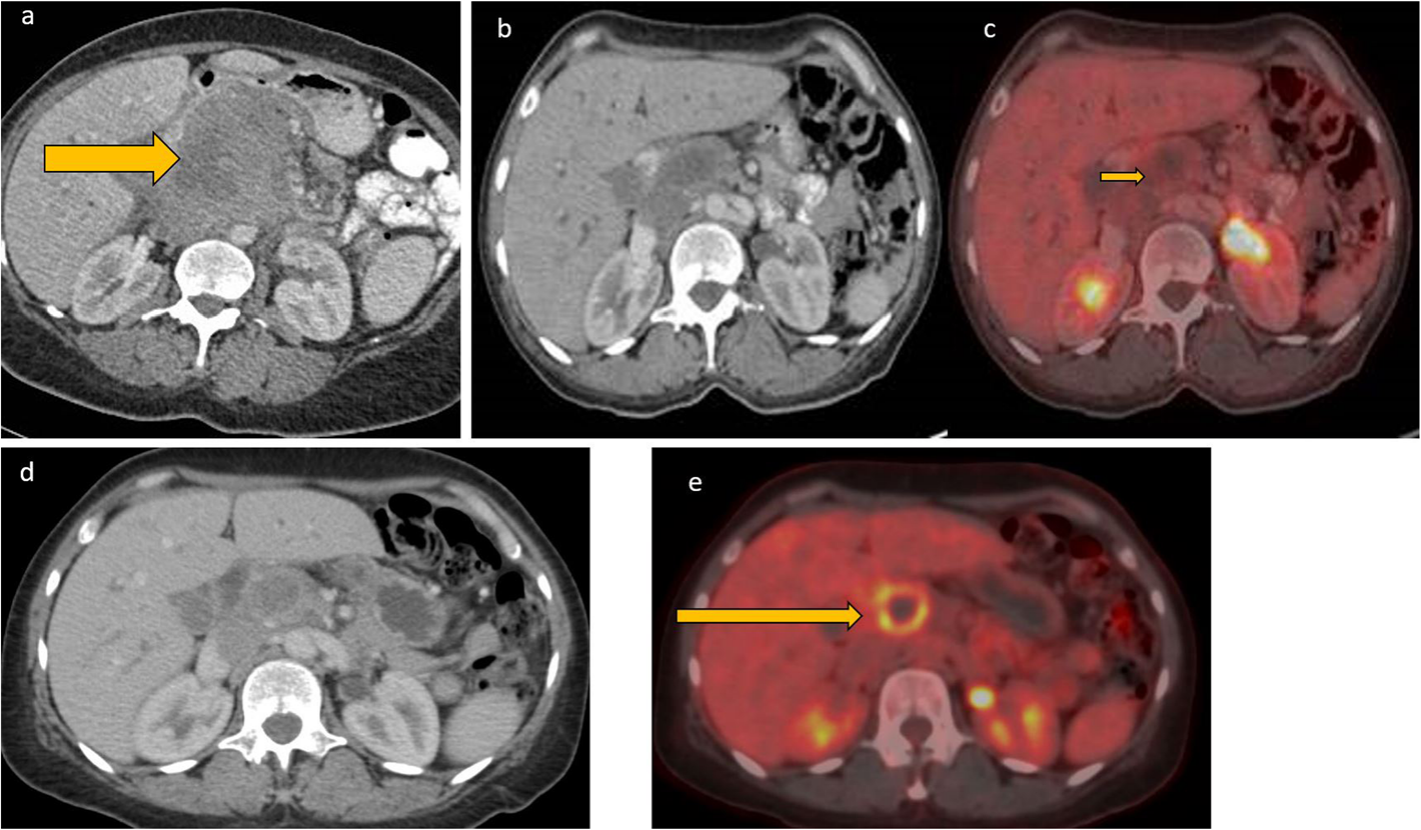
A 46-year-old female patient who had pancreatic lymphoma (a) had a negative PET CT at the end of treatment (b and c). Nine months later, there was local disease recurrence at the pancreatic head on follow-up FDG PET CT scan (d and e)
